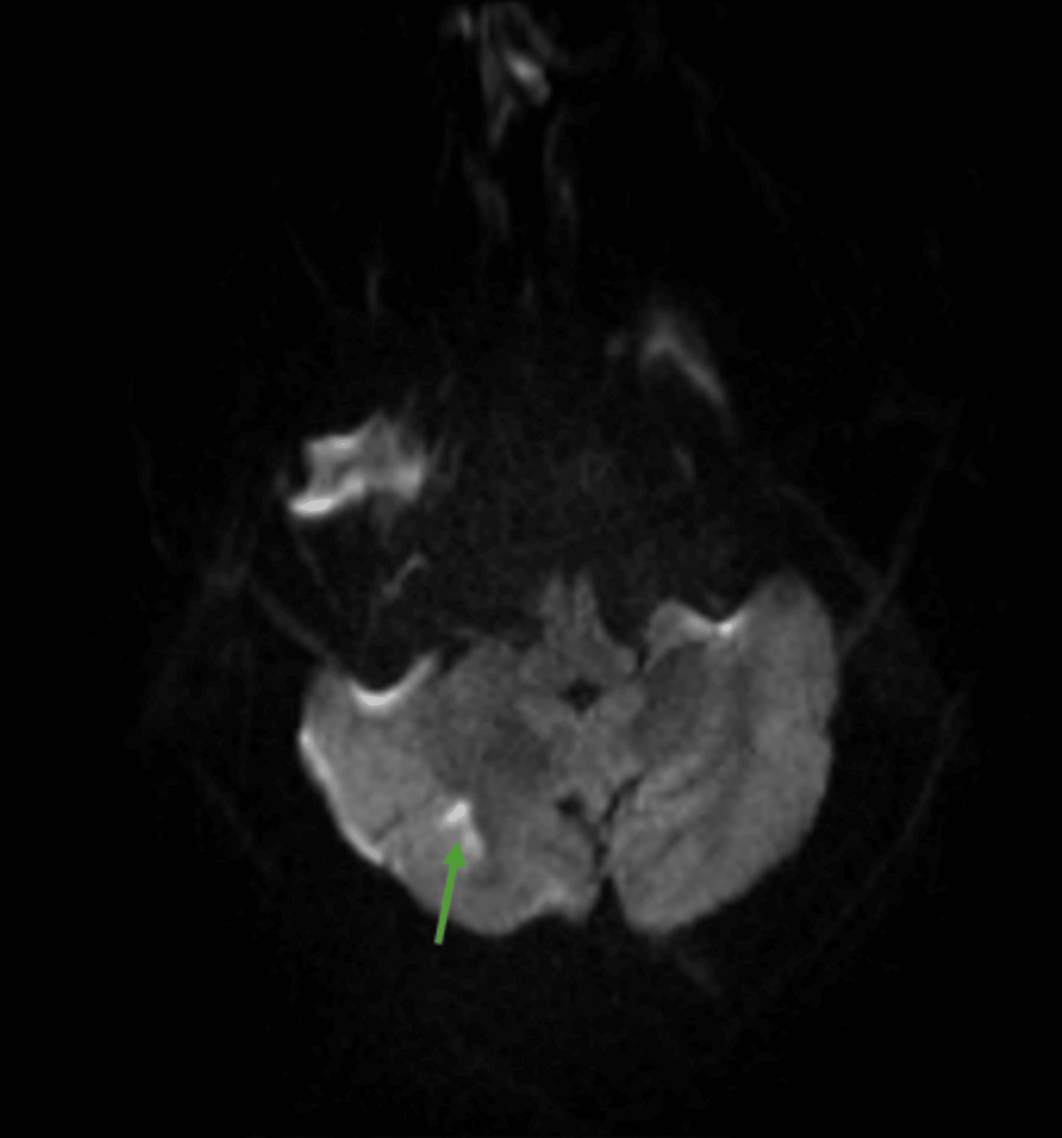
Cerebral infarction on magnetic resonance diffusion-weighted (MRI-DWI) imaging of the brain Diffusion-weighted imaging (DWI) of the brain shows a small focal area of restricted diffusion in the right cerebral hemisphere (green arrow), consistent with an acute ischemic infarct.
